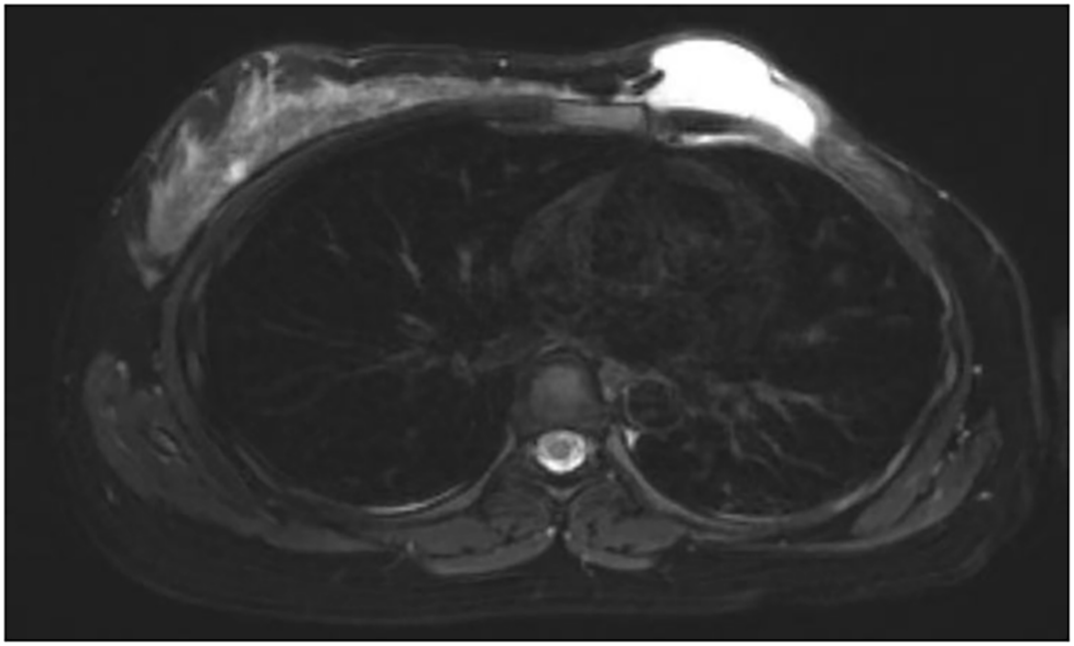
Preoperative breast MRI imaging. Transverse T2 revealed a left chest wall mass suggesting recurrence of a phyllode tumors of the breast, classified as BI-RADS 4C, with invasion into the chest wall and close proximity to the pleura.
